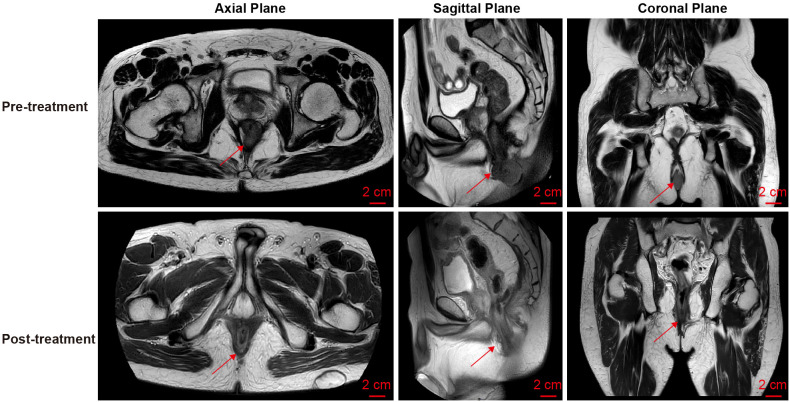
Pelvic MRI images of the metachronous rectal tumor before and after treatment. Arrows indicate the anal canal tumor before treatment (upper row) and after completion of total neoadjuvant therapy combined with intratumoral H101 (lower row).
